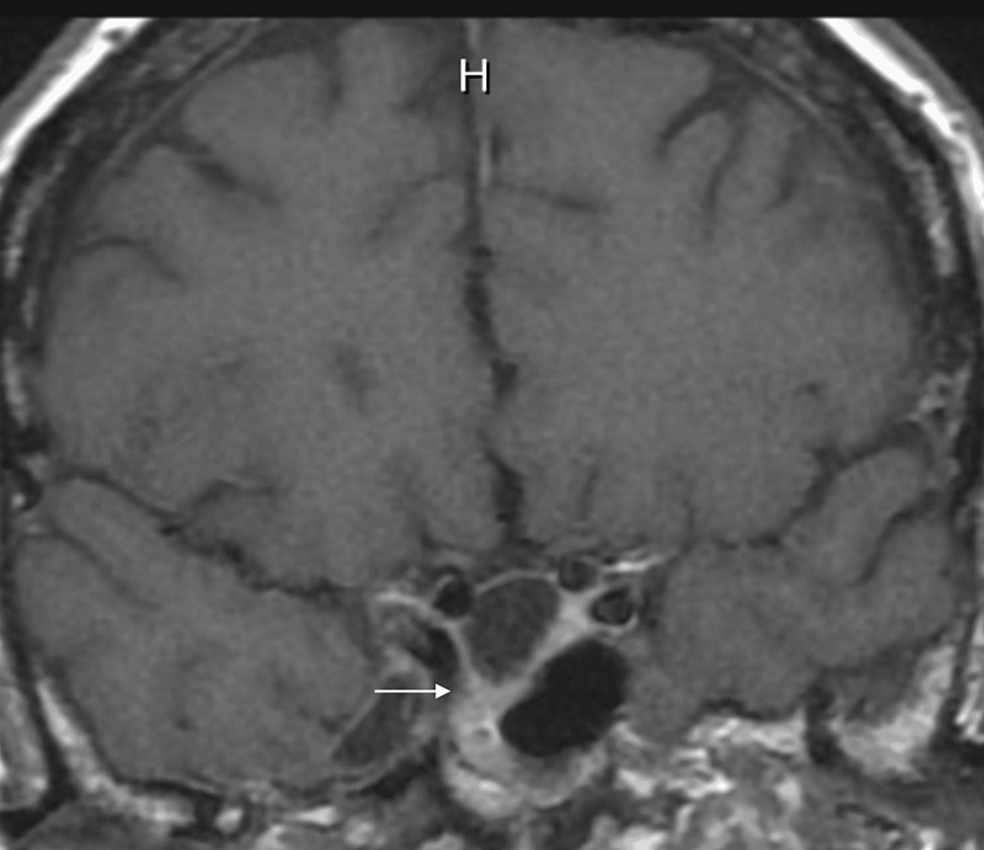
MRI of the sella turcica showing regression of adenoma in the right sphenoid sinus
